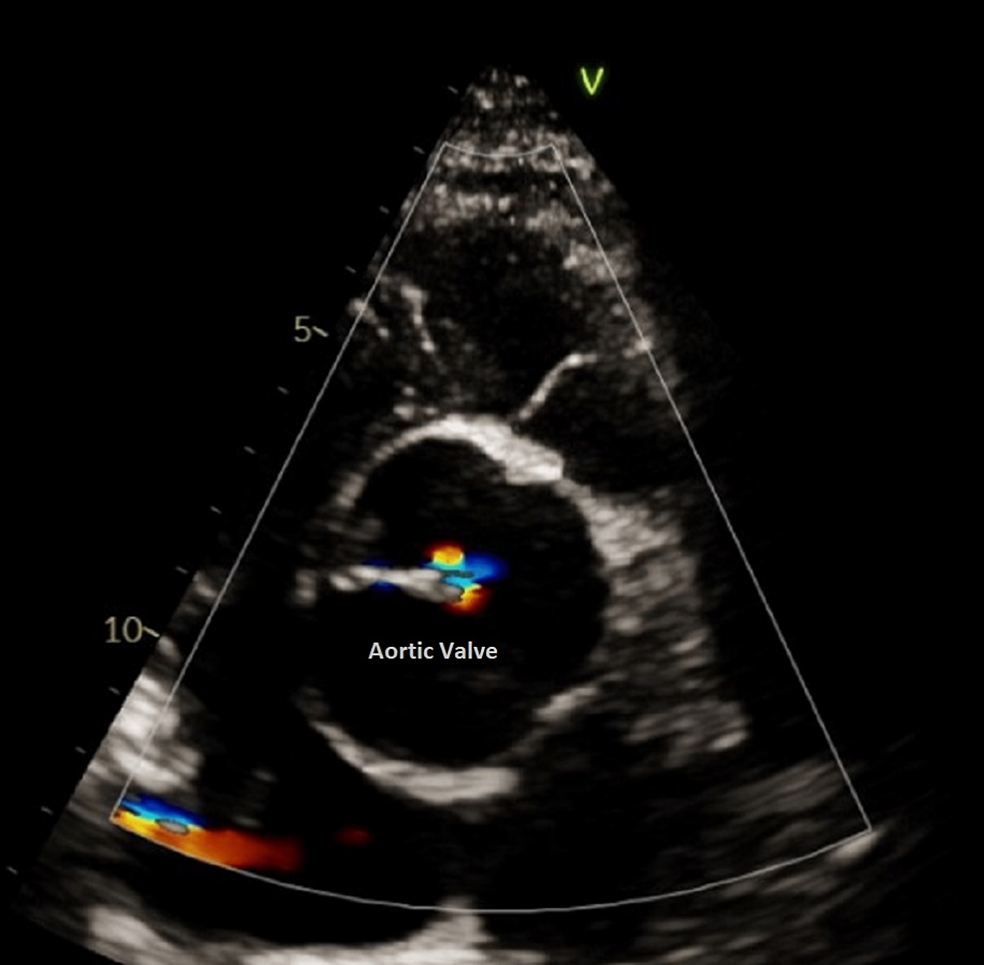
Echocardiogram revealing poor cooptation of the tricuspid aortic valve due to secondary aortic regurgitation
